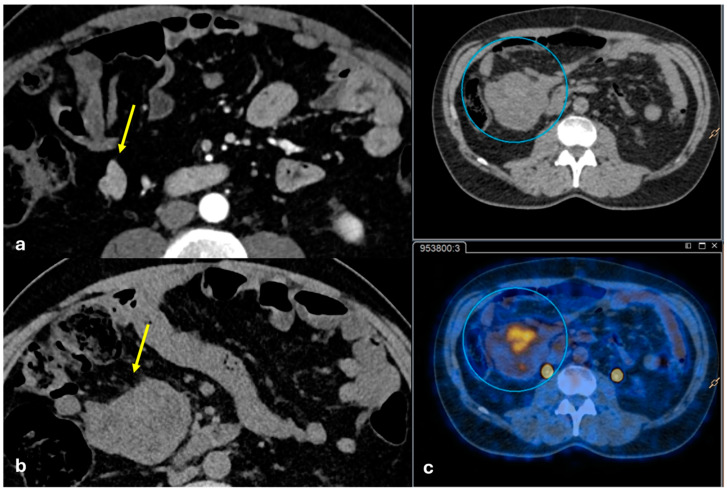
Peritoneal sarcomatosis. A middle-aged man. CECT was made during follow-up after GIST removal. In the first image, ((a), arrow) a hypervascular solid nodule is depicted in the mesentery. After 6 months, the same nodule became larger (CT (b), arrow) and showed FDG uptake at PET-CT (c).
